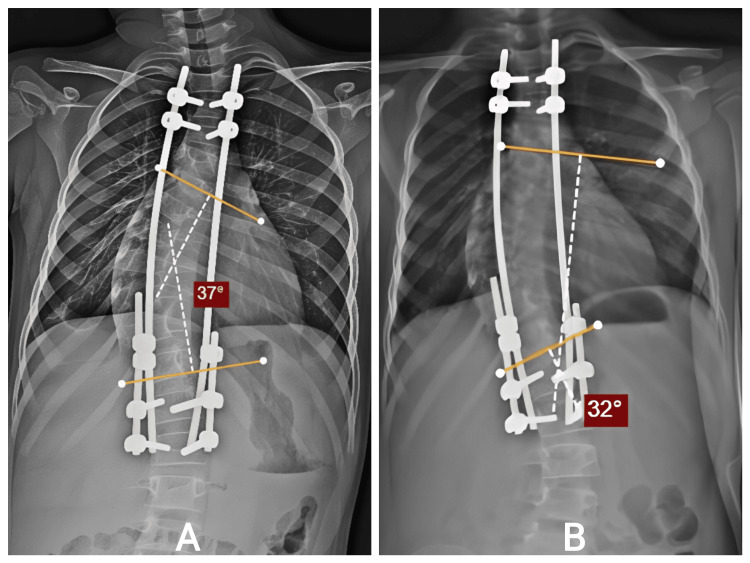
Follow-up Distraction Radiographs. A: After T3–L2 dual growth rods: Cobb reduced 82.4° → 37°, thoracic height 162 → 180 mm, kyphosis 41° → 37°. B: 32°, thoracic height 195 mm. T3: thoracic vertebra; L2: lumbar vertebra; mm: millimeters.
